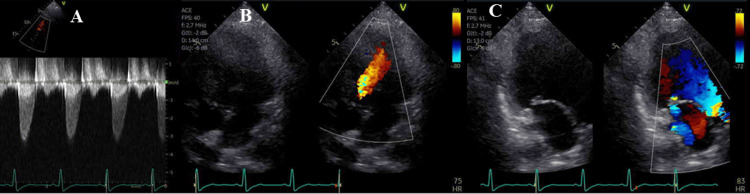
Preoperative transthoracic echocardiography Figure 1A shows the aortic valve velocity. The maximum aortic valve velocity was 3.2 m/s, and the estimated maximum pressure gradient was 41 mmHg. Figure 1B shows aortic regurgitation with the regurgitant jet reaching the apex. Figure 1C shows atrioventricular valve regurgitation. The regurgitant jet is slightly eccentric and directed posteriorly.
